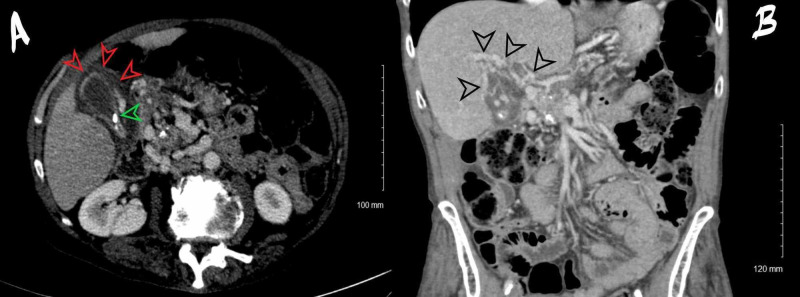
CT scan. (A) Axial CT scan through the gallbladder; gallbladder: two gallstones are present (green arrow). The gallbladder is not overly distended. There is pericholecystic fluid present (red arrows); pancreas: the pancreas is atrophic with parenchymal calcifications and prominence of the pancreatic duct. (B) Coronal reconstruction CT scan through porta hepatis shows normal caliber bile ducts. There is a cavernous transformation of the portal vein (black arrow). Numerous varices are identified, including perigastric and pericholecystic.
